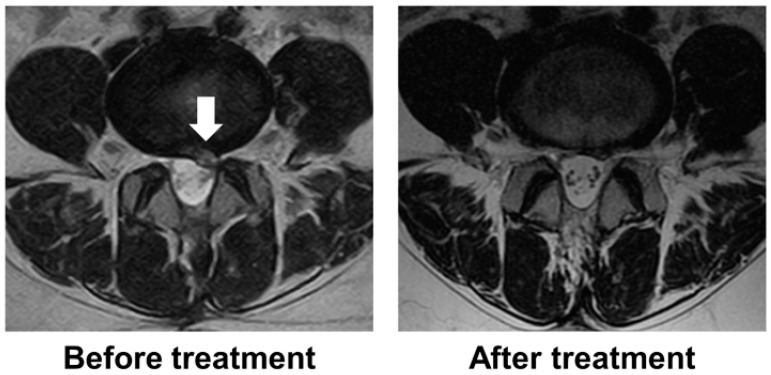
Representative magnetic resonance images before and 3 months after the treatment of a patient with herniated disc resolved by condoliase treatment. A white arrow indicates a herniated disc.
